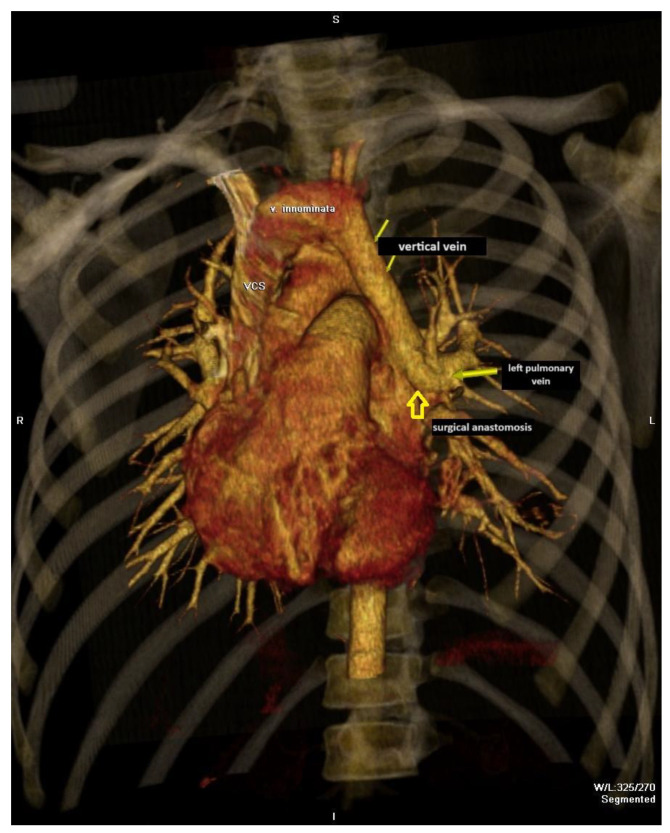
A 3D reconstruction of the CT angiography in the patient with persistent vertical vein after total anomalous pulmonary vein return surgery.
